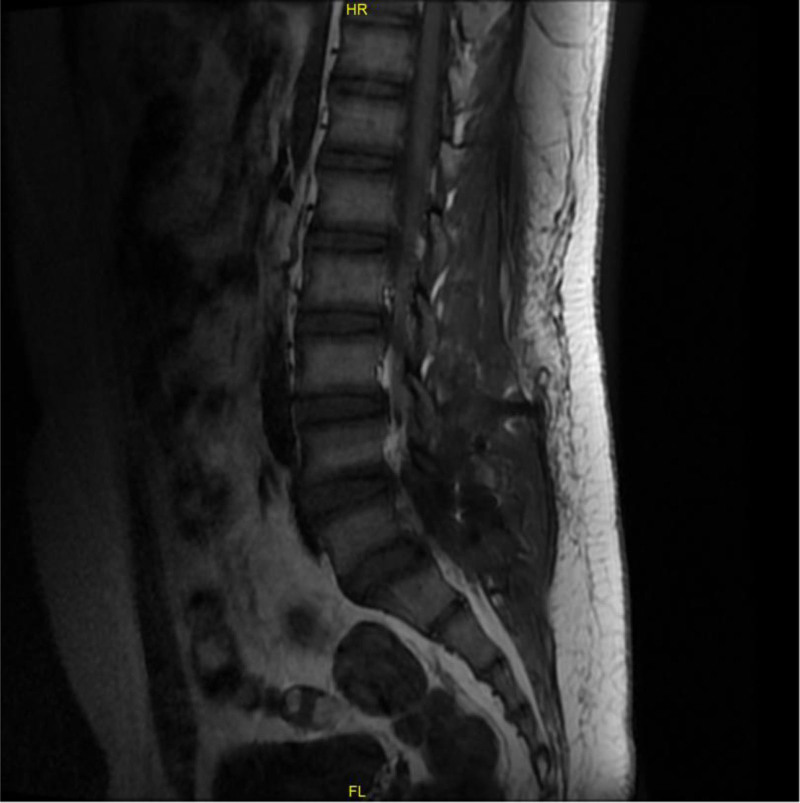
During follow-up, lumbar MRI examination showed postoperative changes, with L5 anterior spondylolisthesis and L4/5, L5/S1 disc bulging. MRI = magnetic resonance imaging.
